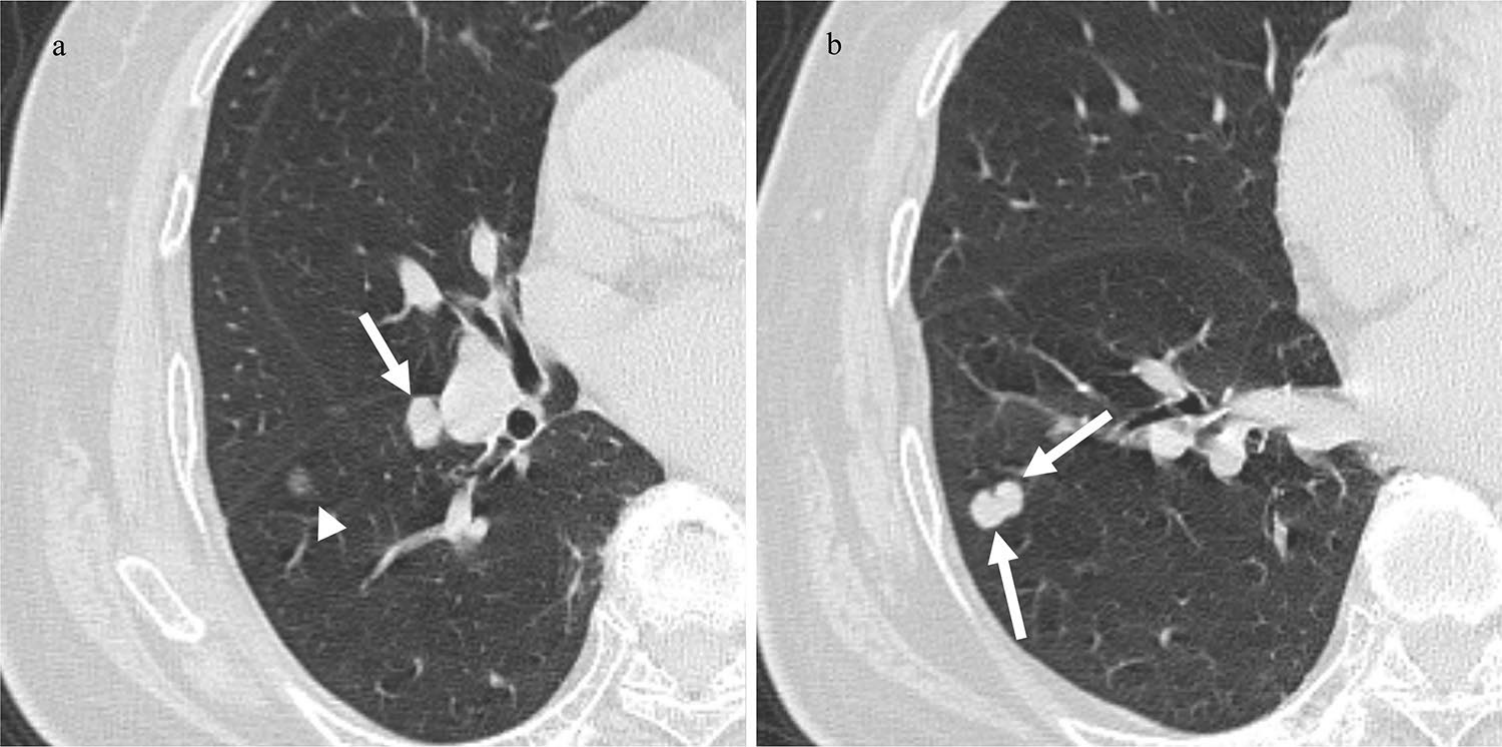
An 84-year-old woman with acral melanoma with a solid nodule-dominant pattern. a, b. Follow-up CT 9 months after the operation shows multiple metastases as solid nodules in bilateral lungs (white arrows). a. Some metastases appeared as GGNs (white arrowheads). After 5 months, some of the GGNs became solid nodules (not shown)
